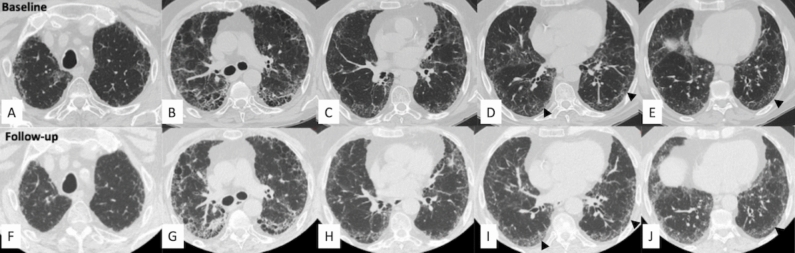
Disease progression, assessed through a side-by-side comparison of high-resolution computed tomography (HRCT) examinations in a patient with PPF secondary to myositis. Baseline HRCT scans (A–E) and follow-up HRCT scans (F–J) were evaluated considering imaging features of five thoracic levels: ground-glass opacifications and reticulations are slightly more represented in the basal regions (black arrowheads). In the assessment of fibrotic disease progression, a side-by-side comparison is strongly recommended in order to reduce variability and to increase reproducibility among radiologists and clinicians
